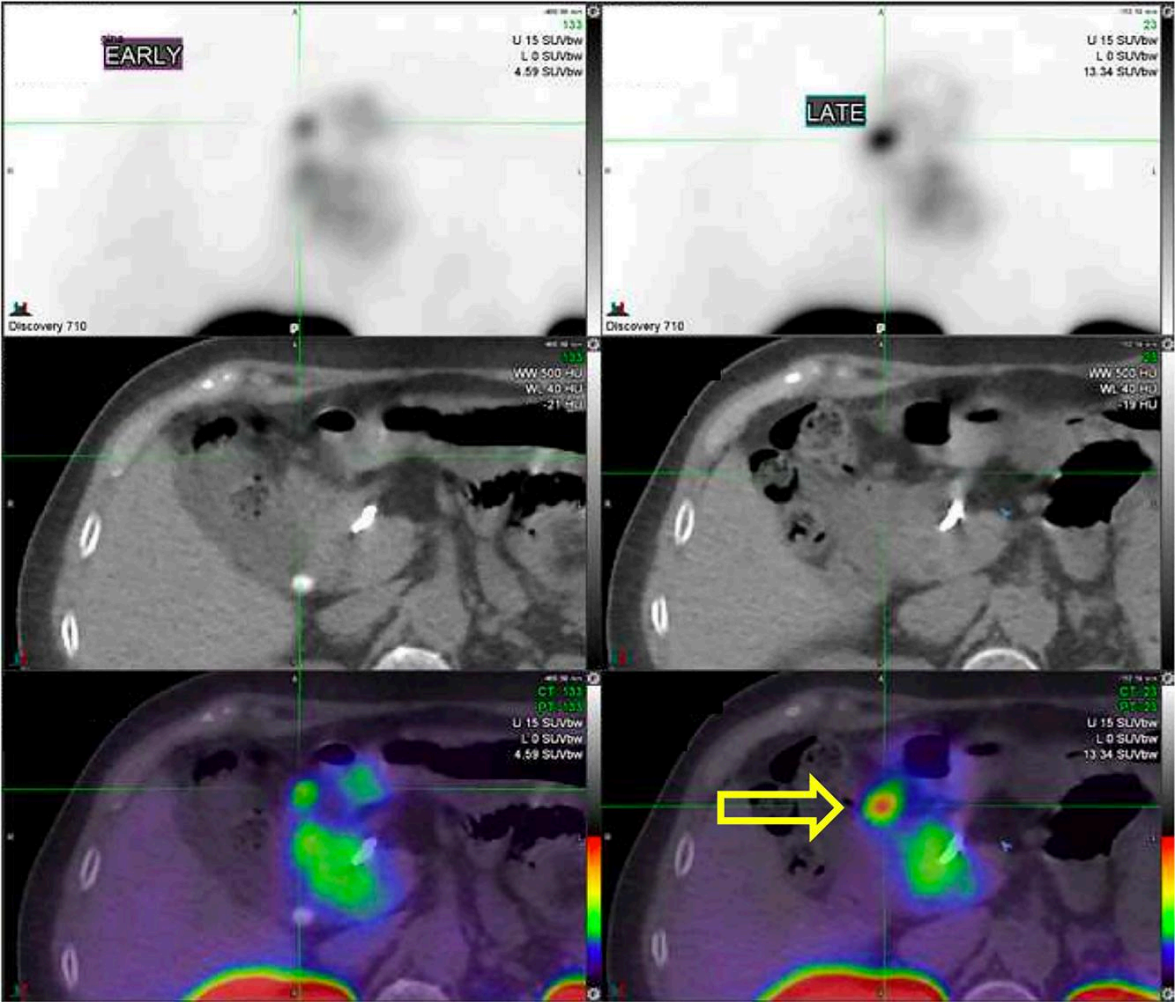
Apparent localization of insulinoma (arrow) with Ga-68 GLP-1 PET-CT.
